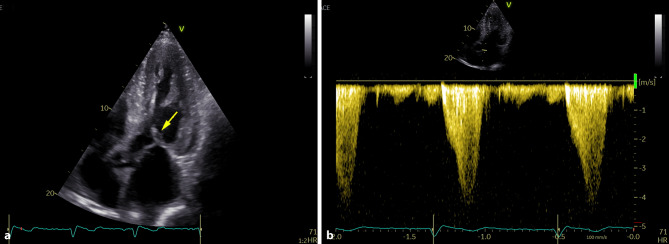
Echocardiography: a apical five-chamber view demonstrating systolic anterior motion of the anterior mitral valve leaflet (*yellow arrow*) causing dynamic left ventricular outflow tract obstruction; b continuous wave Doppler tracing showing typical “dagger-shaped” velocity signal of dynamic left ventricular outflow tract obstruction with resting gradient of 70 mm Hg
